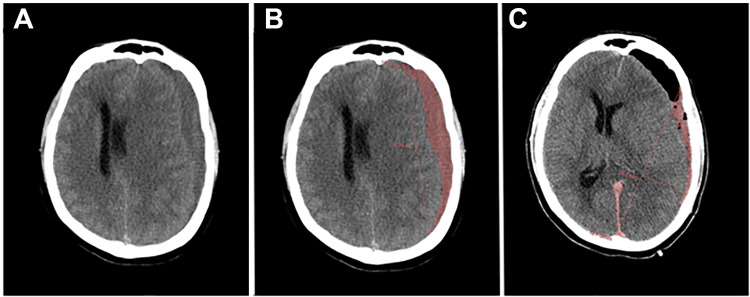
Axial CT images of a patient with chronic SDH (A) Preoperative and (B) preoperative with hematoma volume highlighted in red using Philips Multi-Modality Tumor Tracking software. (C) Postoperative with hematoma volume highlighted.
